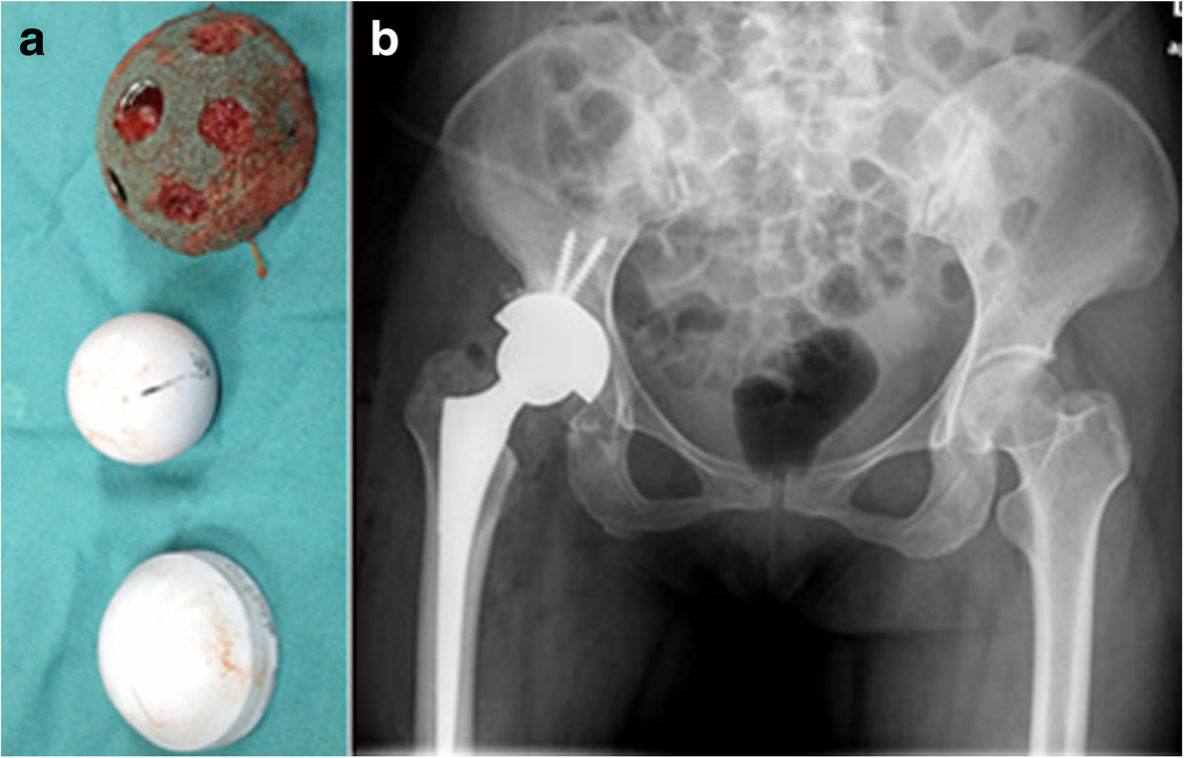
Hip revision in the foregoing patient at 6 months postoperatively. A securely fixed acetabular component was confirmed during the revision surgery. Bone ingrowth was shown within the surface of acetabular component (a). b A trabecular metal acetabular component with decreased anteversion was shown on the radiograph after hip revision
